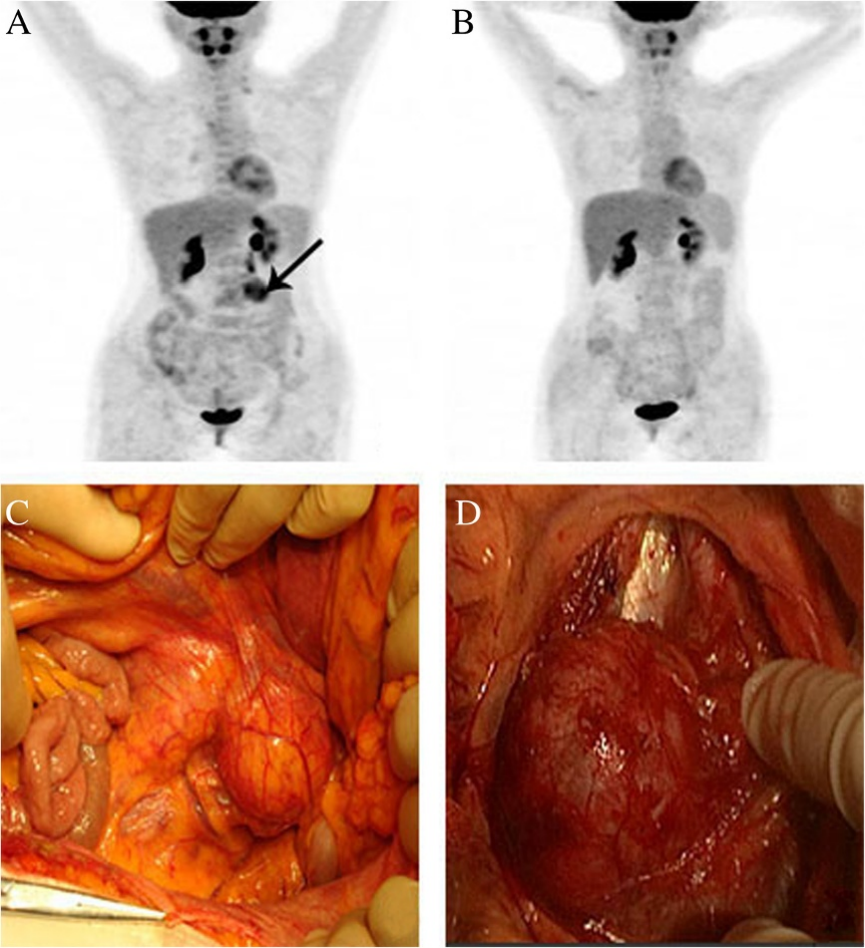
PET-CT scan and macroscopic findings in a recurrent patient (case 11). (A) PET-CT scan before treatment; a black arrow points to the metastatic tumor in the left portion of the fourth lumbar vertebra. (B) PET-CT scan after treatment. (C) and (D) show the retroperitoneal tumor fused by several para-aortic lymph nodes.
